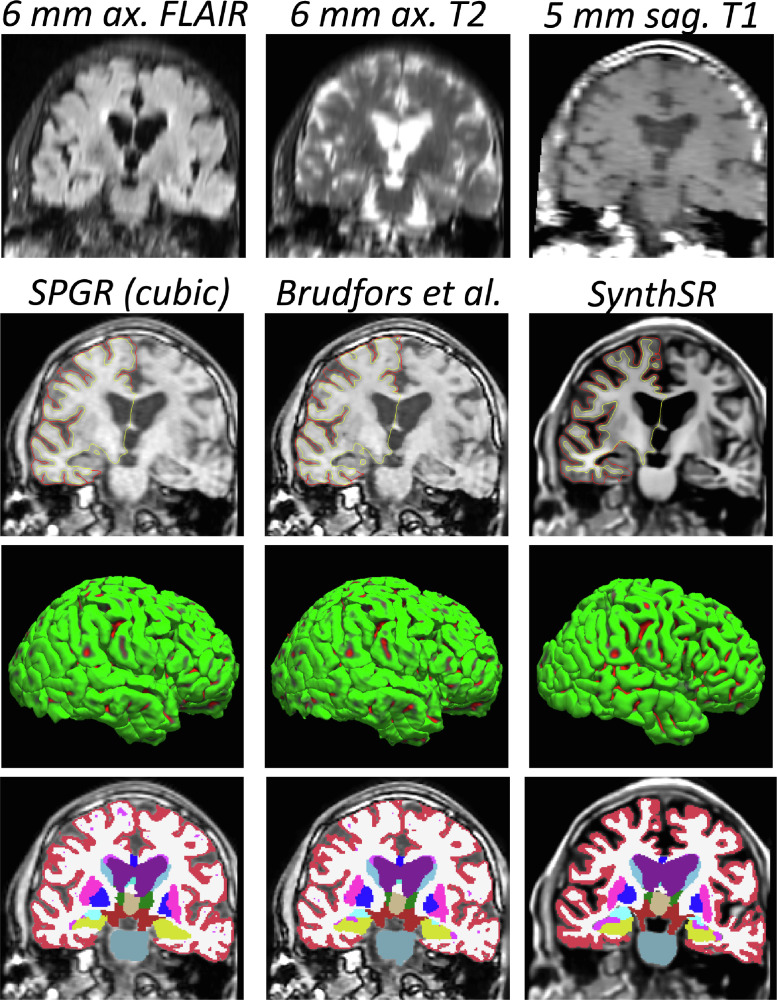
Joint SR / synthesis of an exam from the MGH dataset. The top row shows a coronal slice for the FLAIR, T2 and T1-TSE sequences, with cubic interpolation. The second row shows the corresponding T1-SPGR slice, along with the SR volume produced by Brudfors et al. (2018) and the output from our method, with the pial and white matter surfaces of the right hemisphere computed with FreeSurfer 7. The third row shows the 3D rendering of the pial surfaces. The bottom row shows the volumetric segmentation obtained with FreeSurfer 7.
